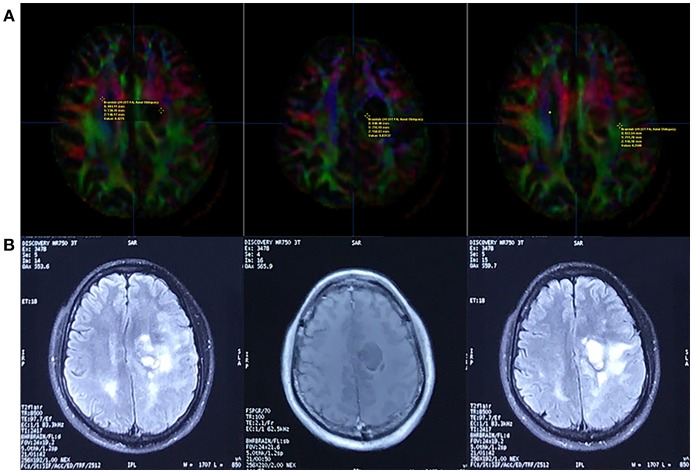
Fractional Anisotropy (FA) parametric map based on DTI (A) and corresponding FLAIR/contrast-enhanced T1-weighted volume images (B). The FA value of the contralateral normal white matter, non-enhancing tumor core, and edematous brain was 0.4275, 0.03137, and 0.2588, respectively (line A, from left to right).
